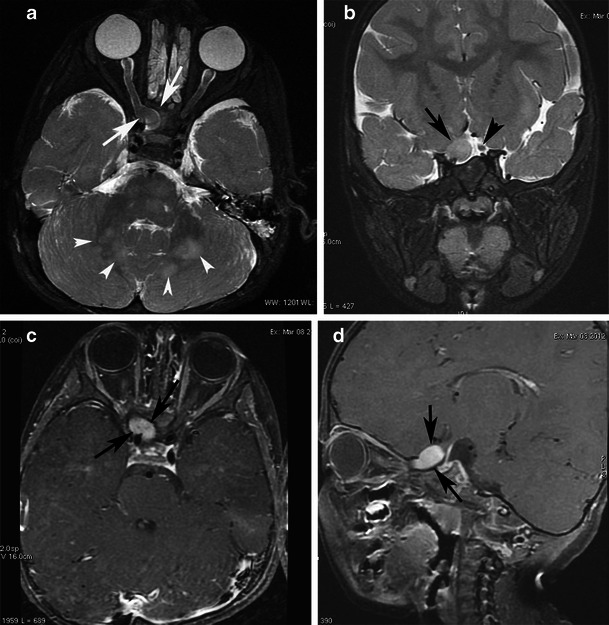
A pilocytic astrocytoma of the optic nerve in a 3-year-old girl with NF1. a An axial T2-weighted image reveals characteristic kinking of the enlarged right optic nerve within the intraconal compartment and enlargement (arrow) of the posterior extraconal compartment. Note the characteristic NF1 bright lesions in the cerebellar hemispheres (arrowheads). b A coronal STIR image shows the enlarged right optic nerve (arrow) in comparison with the normal left optic nerve (arrowhead). On these contrast-enhanced axial (c) and sagittal (d) T1-weighted images, intense enhancement of the extraconal component is observed (arrows)
